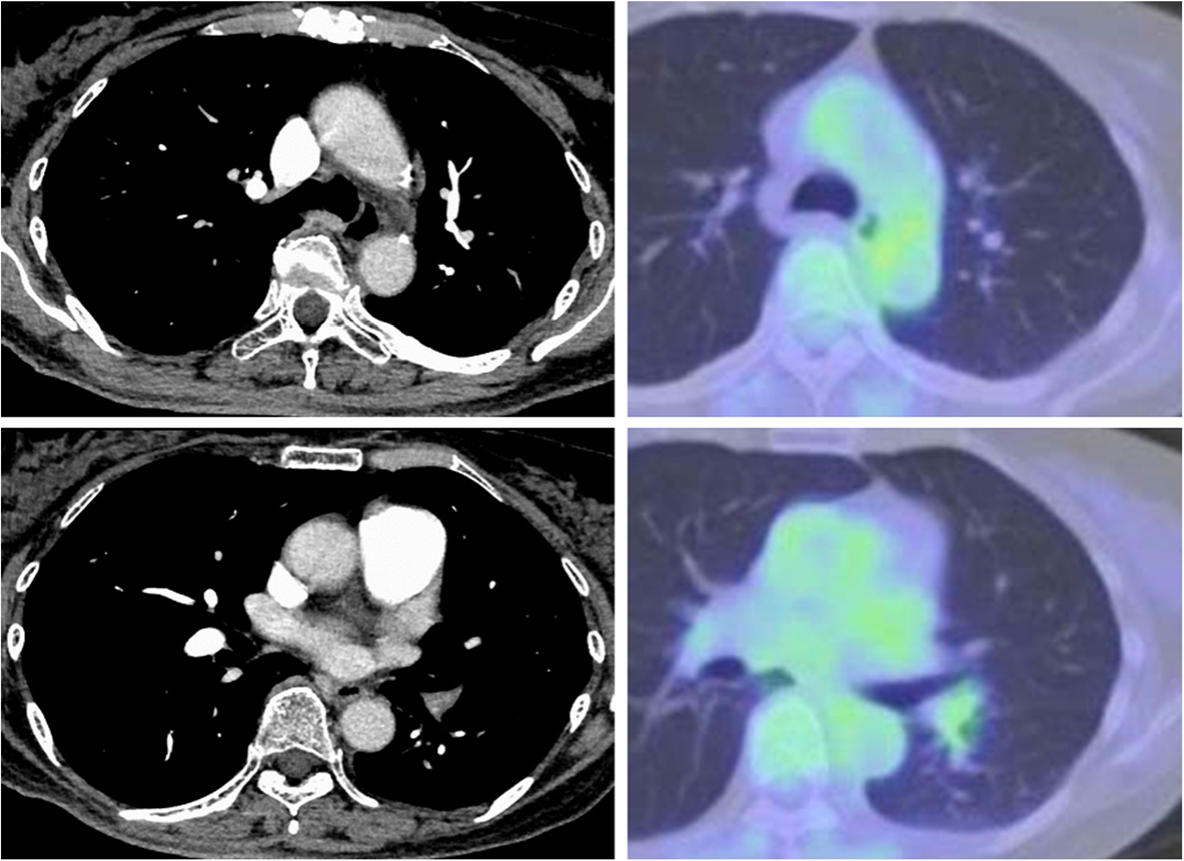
Computed tomography and positron emission tomography after the treatment with alectinib
